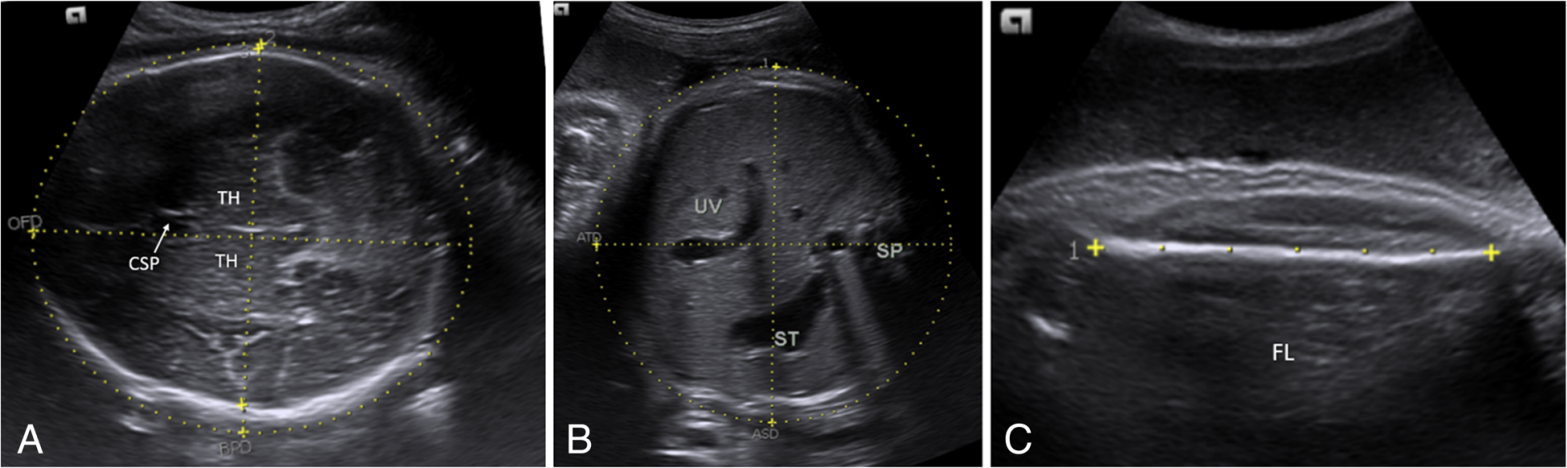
Sonographic parameters used to estimate fetal weight. B demonstrating the fetal head at the level of bithalamic (TH-thalamus), cavum septum pellucidum (CSP) and third ventricle. At this level was the BPD and HC taken b Abdominal circumference taken at the level of umbilical vein (UV), stomach (ST), spine (SP) and the posterior rib. c demonstrating femoral length measurement
